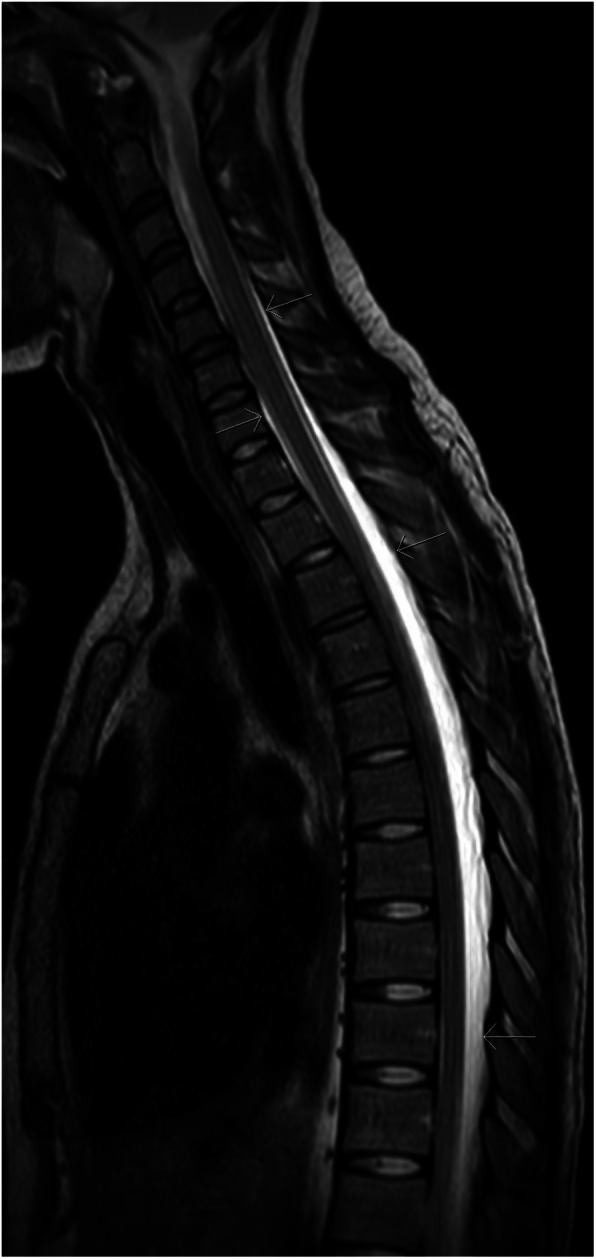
MRI (T2-weighted), upper spine, sagittal: Accumulated CSF (bright)
